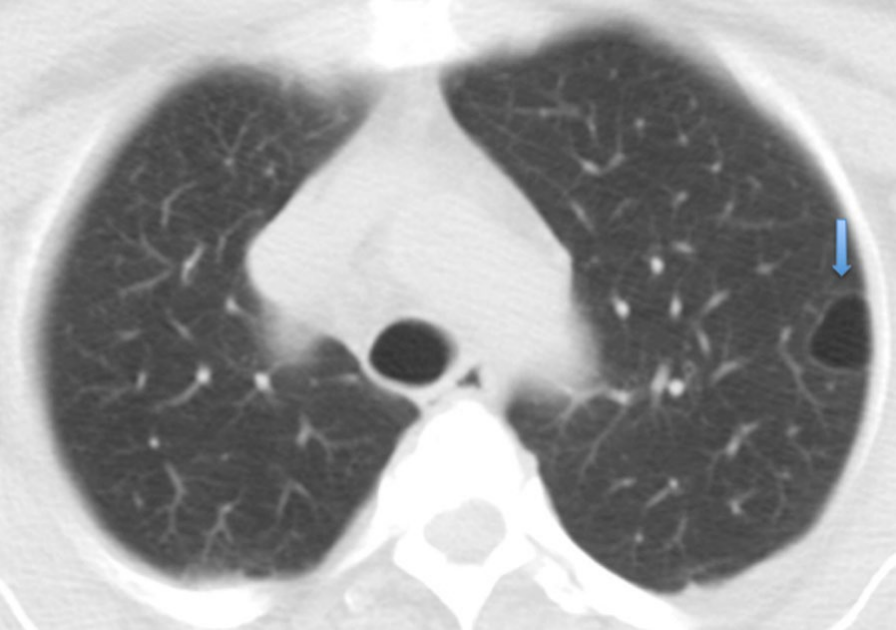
Axial CT of the Chest: Pulmonary window. A solitary thin walled lung cyst was present in the periphery of the left upper lobe (*arrow*)
